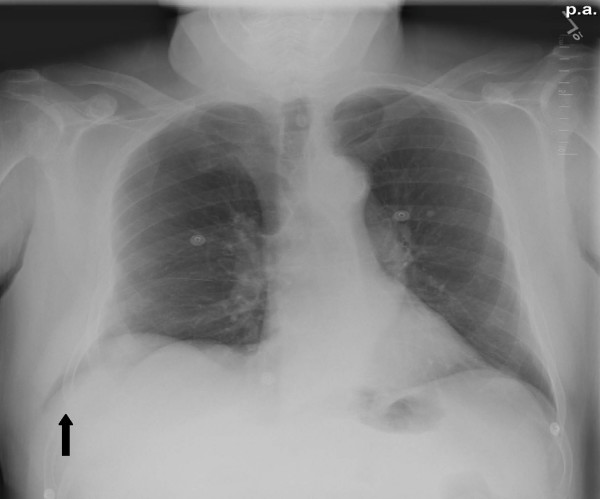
Chest Radiograph shows lung tissue outside thoracic cavity.
